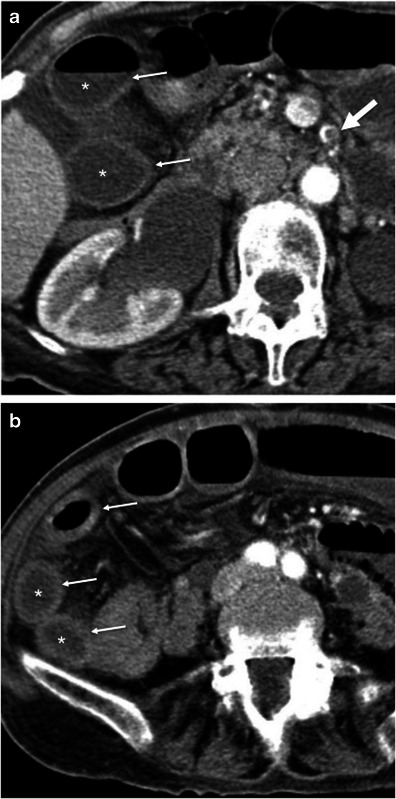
Grey attenuation pattern in small bowel ischaemia. Axial contrast-enhanced CT scans show distended fluid-filled small bowel loops (*asterisks* in a and b) with hypoenhancing thick walls (*thin arrows* in a and b) indicating ischaemia in a patient with partial occlusion of the superior mesenteric artery (*large arrow* in a). *Adapted from the electronic poster “Bowel wall thickening—a complex subject made simple” DOI:*10.5444/esgar2011/EE-063
